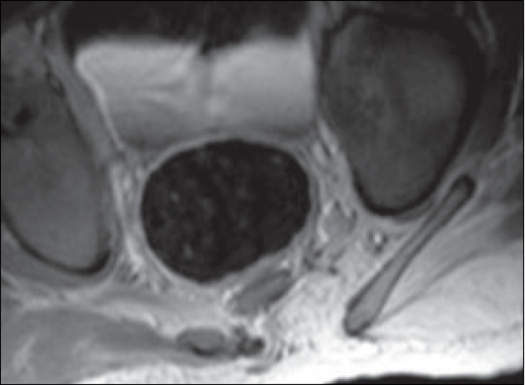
Axial T1W MRI showing the bony strut with spinal dyraphism
